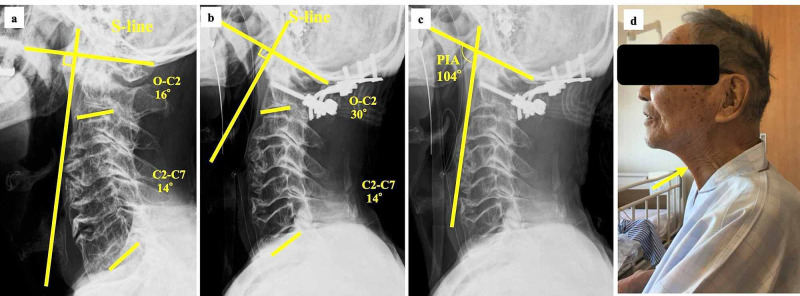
O-C2 angle, S-line, C2-7 angle, and pharyngeal inlet angle Postoperative lateral radiograph (b) showing a larger O-C2 angle, the swallowing line (S-line) at the more anterior position, and no change of the C2–C7 angle compared with the preoperative lateral radiograph (a). Postoperative pharyngeal inlet (PIA) angle measured >90° (c). Postoperative lateral photograph (d) showing hypertonicity of hyoid muscles (arrow) due to cervical hyperlordosis
